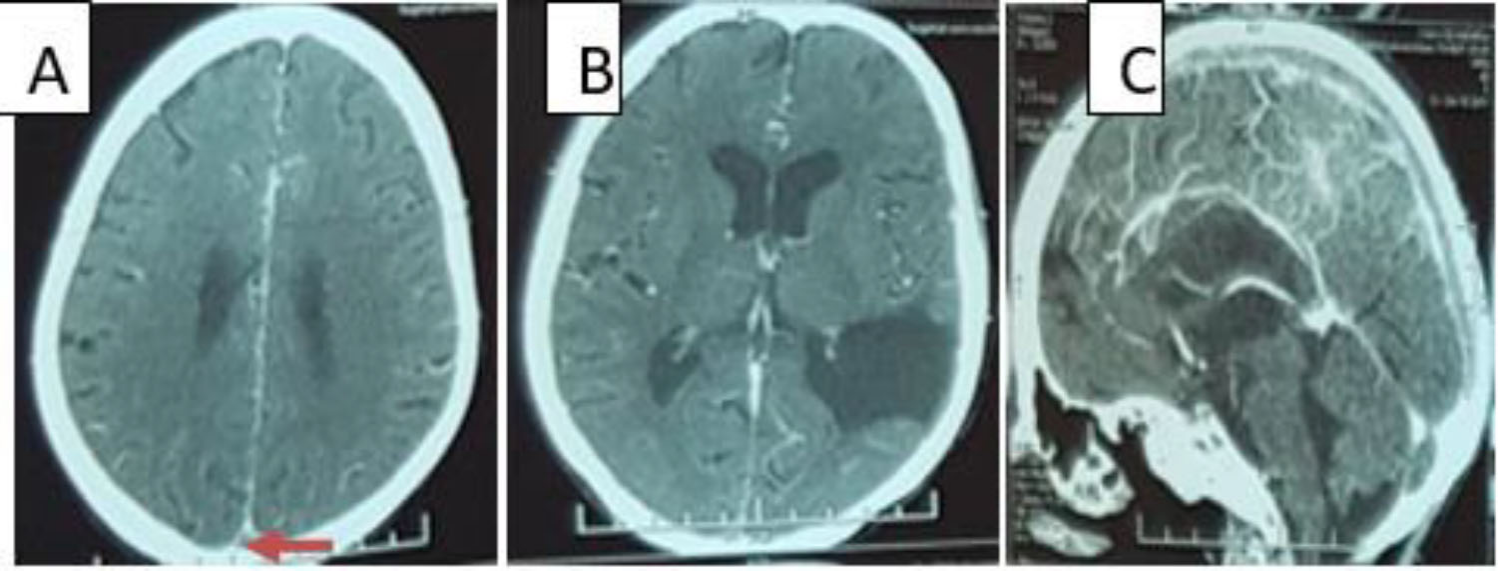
Cerebral CT scan (A, B: axial plane, C: Sagittal plane) showing a venous thrombosis of superior sagittal sinus (A, C) and a left temporoparietal porencephalic cyst (B).
